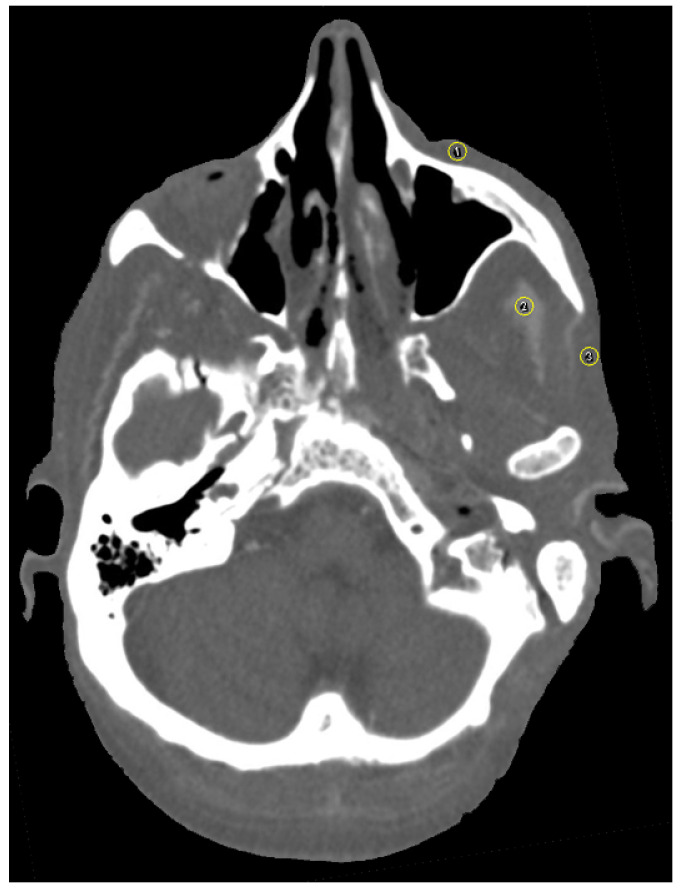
Axial view of selection of three circular regions of interest (ROI) in close proximity to the zygomatic implant using the ROI manager in ImageJ [(1) the zygomatic soft tissue, (2) the temporal muscle and (3) the parotid gland]. Plugins used were CT Window Level and SPICE-CT Package for Computed Tomography QC (Loveland, J.; 2011). HU window was set to soft tissue. Mean HU values were measured for all ROI to determine artifact occurrence.
